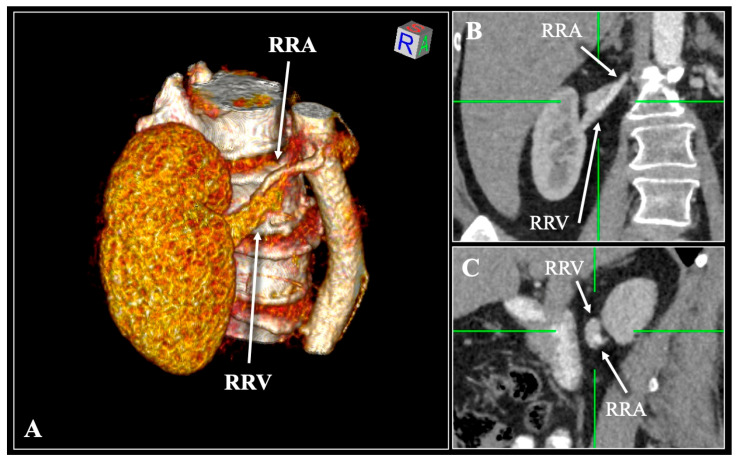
Contrast-enhanced computed tomography (CT) scan of a 63-year-old female patient demonstrating the typical Vein (V)—Artery (A)—Pelvis (P) anatomy. Three-dimensional reconstruction (A) and multiplanar reconstruction with coronal (B) and sagittal (C) slices. A single right renal artery (RRA) and vein (RRV) are entering/exiting the hilum. R—right, L—left, S—superior, I—inferior.
